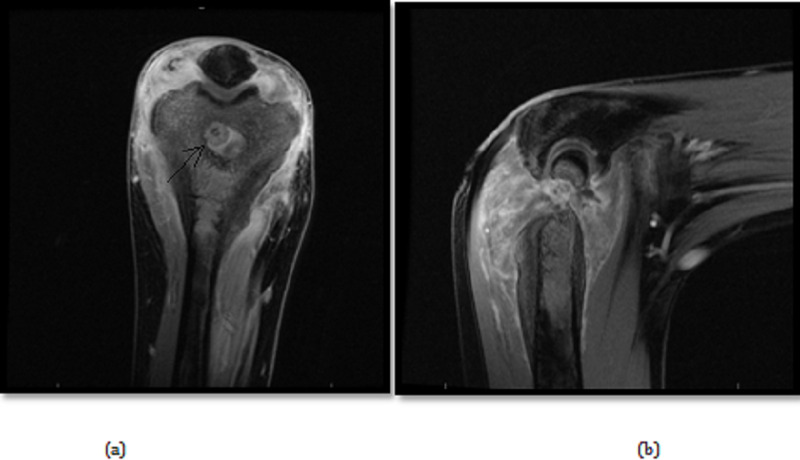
Preoperative magnetic resonance imaging (coronal (a) and sagittal (b), respectively)
